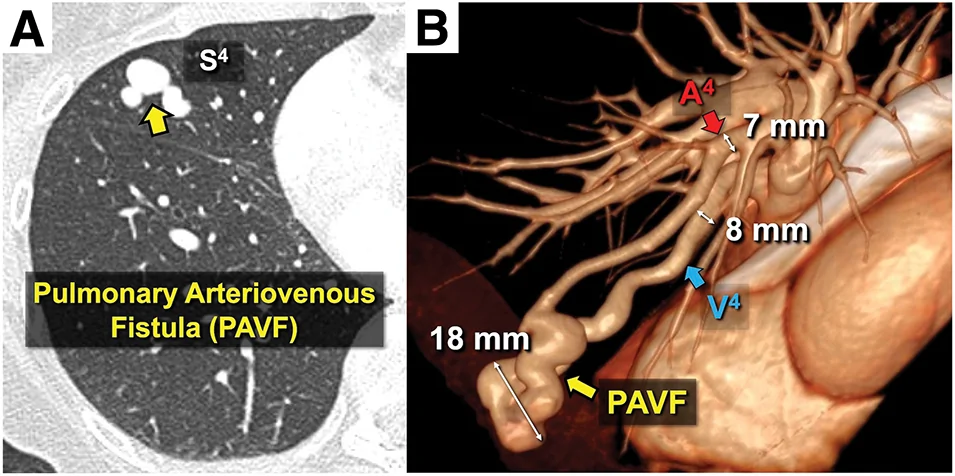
PAVF on preoperative CT and 3D reconstruction images. (A) The PAVF was shown on right S^4^. (B) PAVF was constructed with A^4^ and V^4^, which were dilated to 7 and 8 mm in diameter, respectively. PAVF, pulmonary arteriovenous fistula
